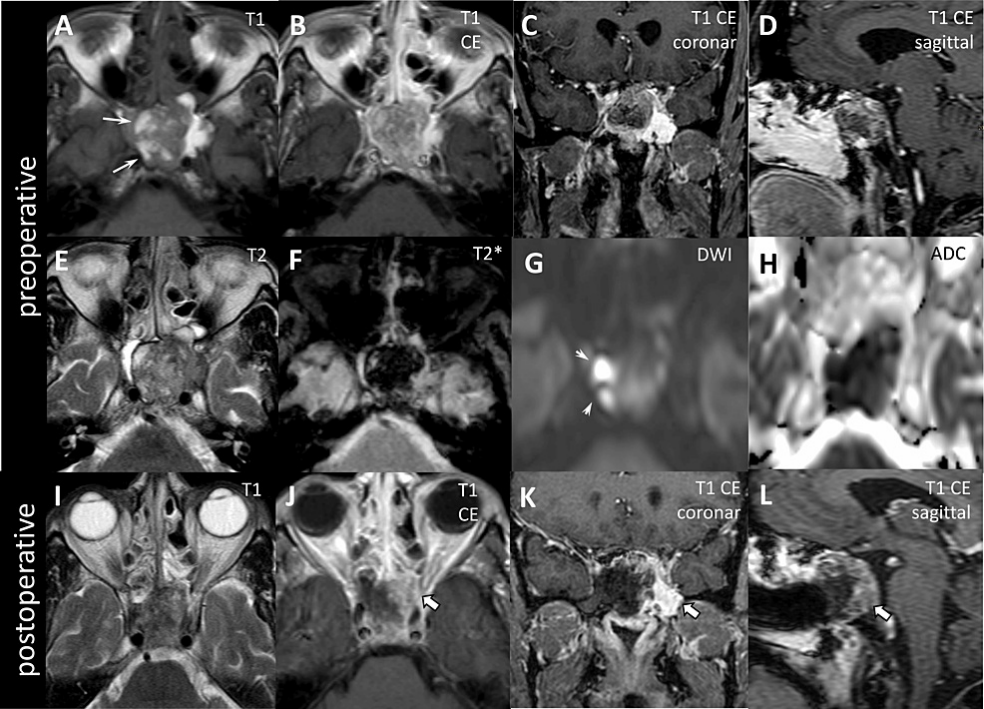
Pre- and postoperative imaging. The figure shows preoperative (A-H) and postoperative (I-L) MR images in axial reconstructions unless otherwise indicated. T1-weighted MRI shows a sellar mass with focal hyperintense spots (arrows) indicating melanin content (A). CE T1 images show inhomogeneous enhancement and infiltration of surrounding structures (B-D). The mass is inhomogeneous on T2 images (E) and has a low signal on susceptibility-weighted images (T2*, F). The melanin-containing areas are diffusion-impaired, as shown by a high signal on DWI (G) and low ADC values (H). Postoperative T1 (I) and T1 CE show residual tumors at the left sella and the left cavernous sinus (block arrows, J-L). MRI: magnetic resonance imaging; CE: contrast-enhanced; DWI: diffusion-weighted images; ADC: apparent diffusion coefficient
